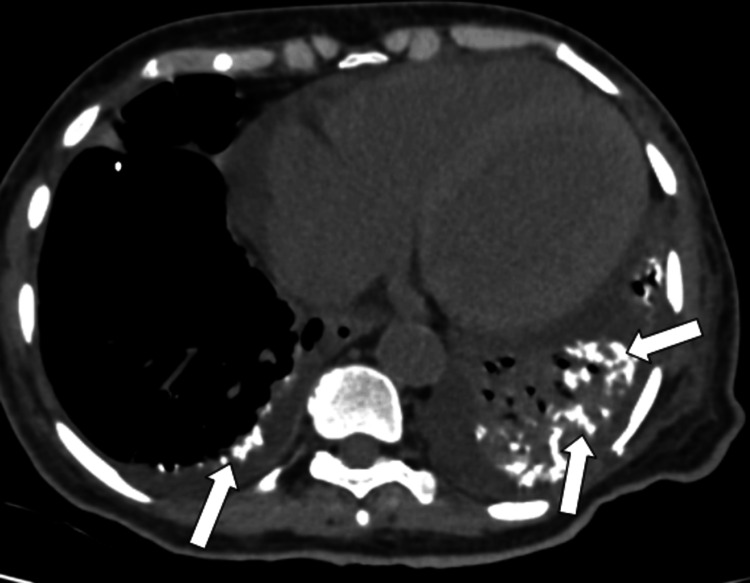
CT axial sections, soft tissue window plain images showing multiple patchy peribronchial calcifications in the left lower lobe and some calcifications along the right lower lobe pleura (indicated by arrows). CT: Computed tomography
